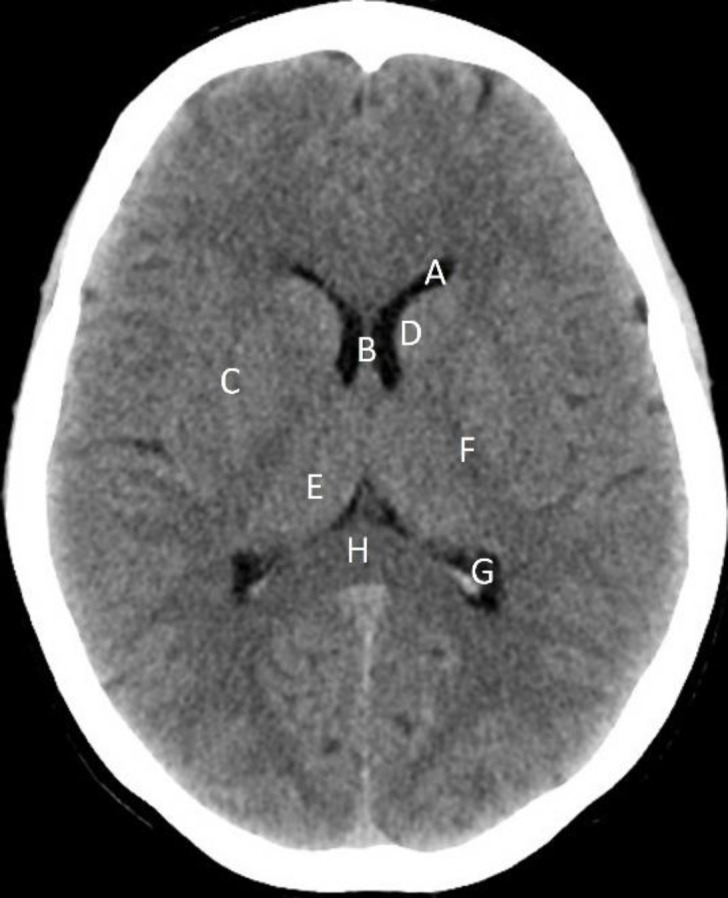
Identify the normal anatomical structures labelled A to H on a non-contrast axial CT brain of a 43 years-old male
